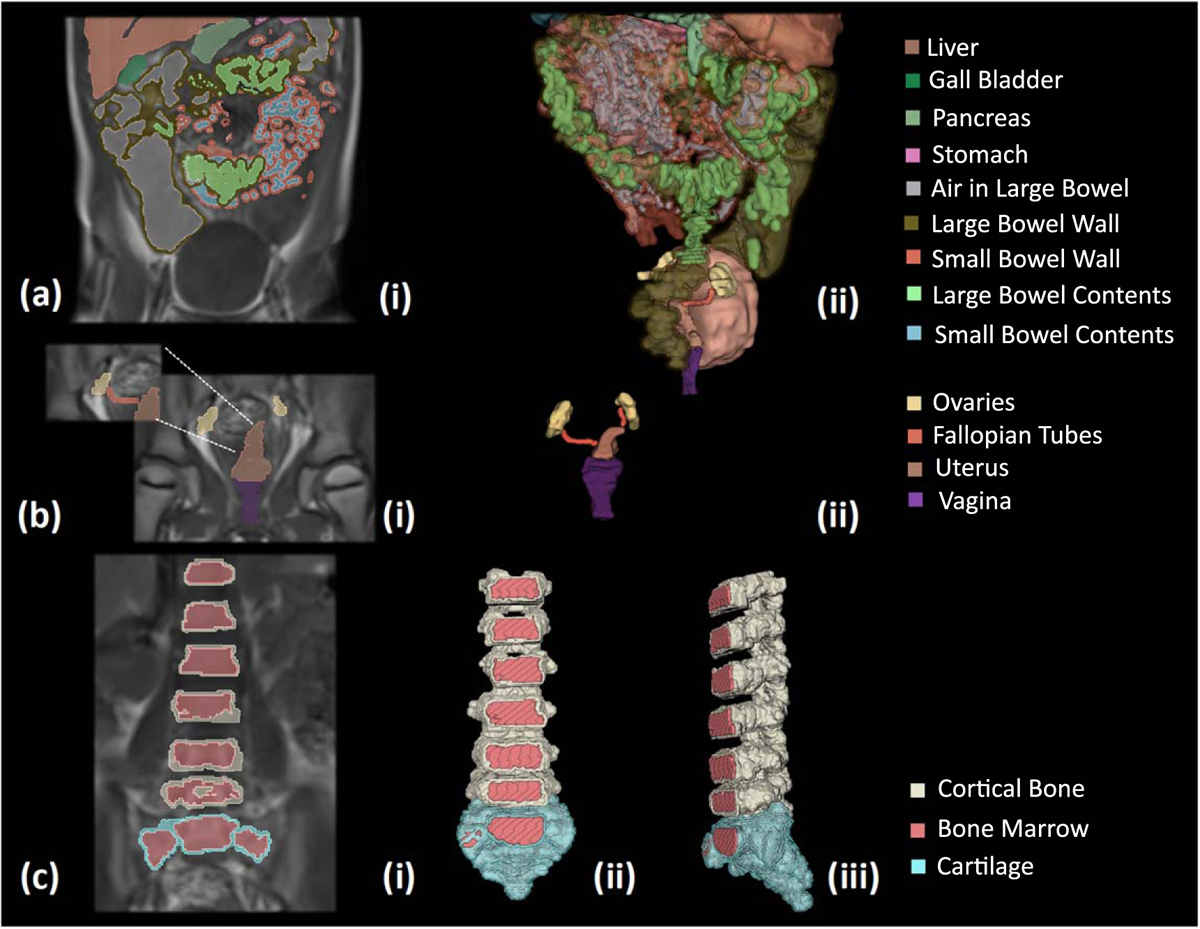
Segmentation and 3D representation of segmented tissues: (a) (i) segmentation of abdominal organs (viscera) on T1 MRI images in coronal plane and (ii) 3D opistholateral view of segmented tissues as reconstructed for the generation of the 3D model. (b) (i) Two different slices that show the segmentation of the female genitalia, including the ovaries, the fallopian tubes, the uterus, and the vagina on coronal MRI images, and (ii) 3D reconstruction of the segmented tissue. (c) Coronal MRI slice with the segmentation of the bone marrow, the cortical bone, and the cartilage of the sacral bone, the lumbar and lower two thoracic vertebrae. Colormap: each color corresponds to different segmented tissue. Not all the segmented tissues were included in this figure, but specific tissues were selected for visualization purposes.
